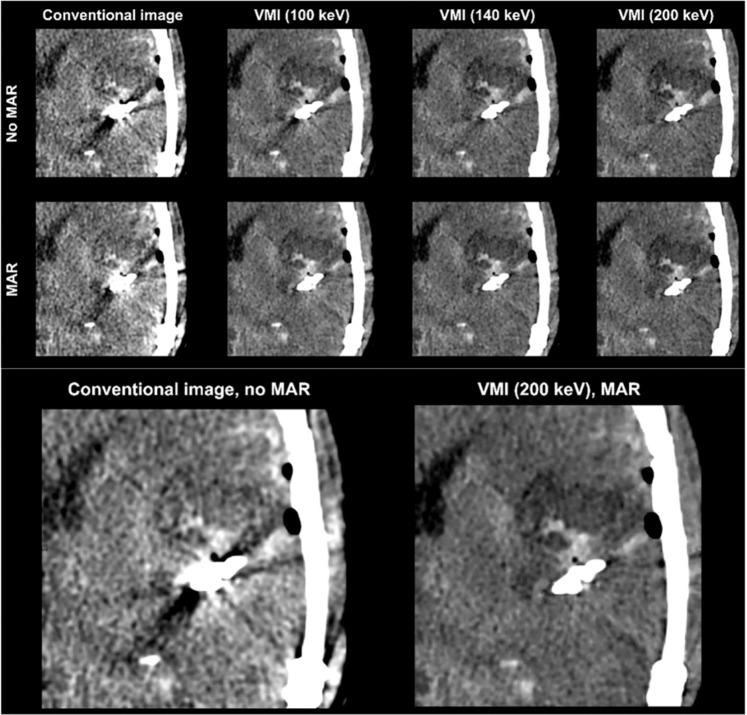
Combination of VMI and MAR completely reduces artifacts and reveals surrounding postsurgical bleeding and brain defect. Conventional CT images (conventional), virtual monoenergetic images (VMI, 100–200 keV), metal artifact reduction algorithms (MAR) and their combination.
